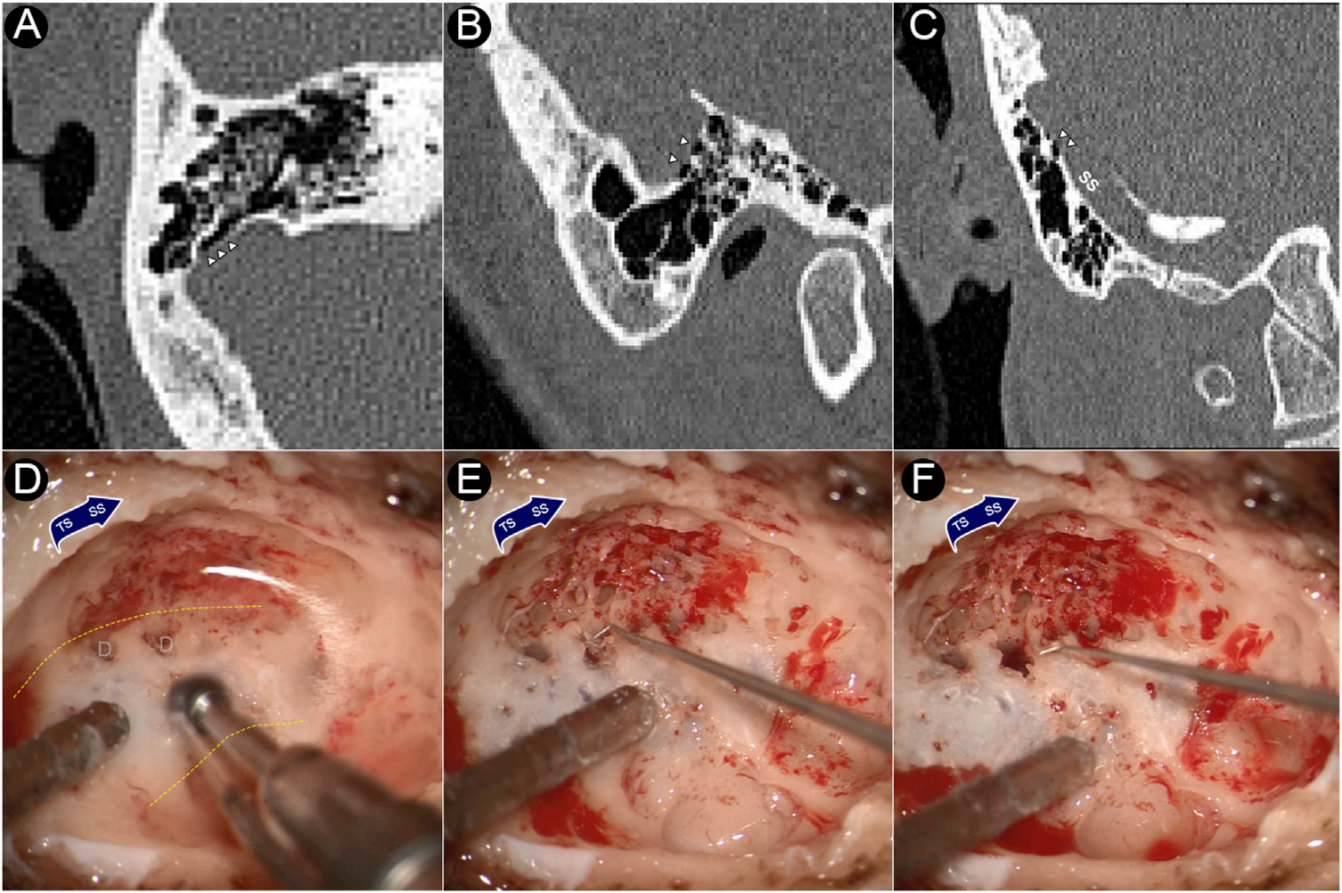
CT images and intraoperative photographs of the participant with sigmoid plate dehiscence. (A) Axial plane of dehiscence; (B) sagittal plane of dehiscence; (C) coronal plane of dehiscence. (D) Dehiscence exposed during skeletonization of sigmoid sinus. (E) Harvesting the dehiscent sigmoid plate. (F) After the removal of the dehiscent sigmoid plate. Triangles indicate the location of dehiscence. D represents dehiscence. Yellow dashed lines indicates the silhouette of the sigmoid sinus.
